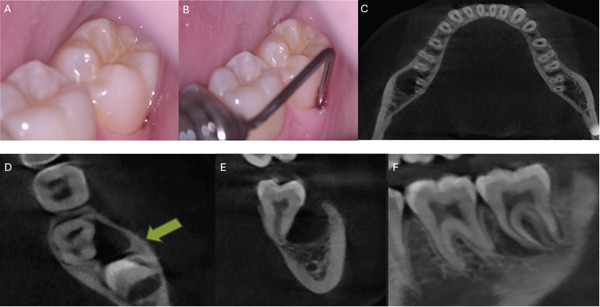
Preoperative CBCT images and clinical photos of Teeth #18 and #19. (A) Photographs of Teeth #18 and #19 at clinical evaluation. (B) The second molar, buccal tilting of the crown, virgin tooth with apparently healthy periodontal tissues. Periodontal probing revealed a 12‐mm furcation (mid‐buccal) pocket consistent with the cyst location. (C–E) CBCT view shows an expansive lesion with a periosteal reaction on the buccal surface, a well‐defined hypodense lesion surrounding the buccal aspect of Tooth #18. The arrow symbol shows an expansive lesion with periosteal reaction on the buccal surface.(F) CBCT sagittal view shows a hypodense lesion involving the mesial root of Tooth #18.
